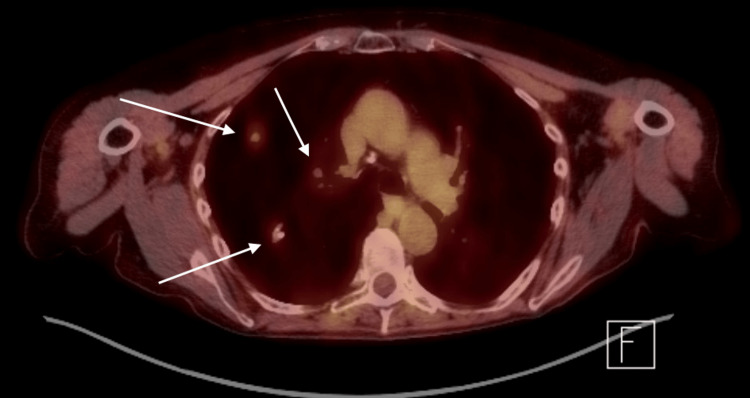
PET scan showing FDG avid lesions in lung fields (white arrows), raising a high suspicion for a neoplastic process PET: positron emission tomography; FDG: fluorodeoxyglucose
